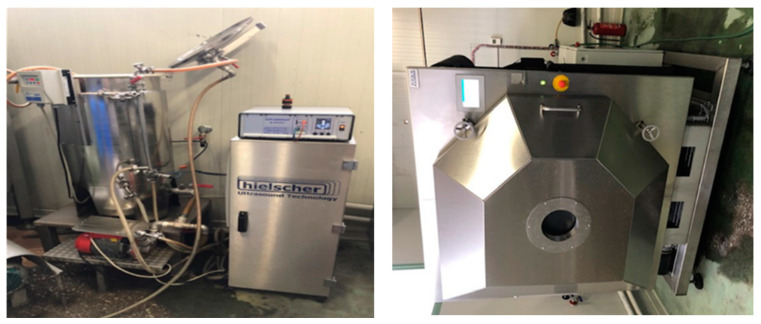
Industrial ultrasound homogenizer, Hielscher model UIP1000hdT (left) and industrial freeze dryer Zirbus GmbH model EKS 100-109 (right).
